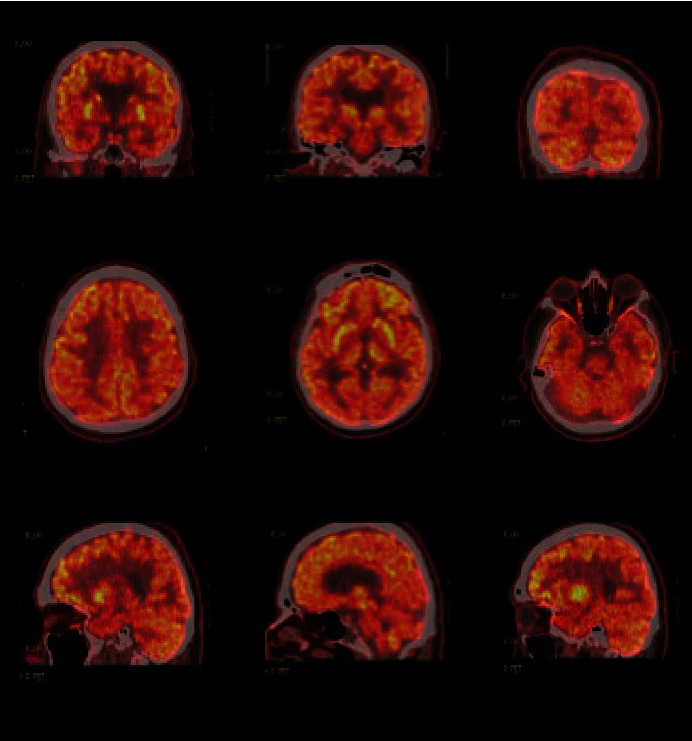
Fluorodeoxyglucose-positron emission tomography (FDG-PET) images of the patient demonstrating hypometabolism of the occipital and temporal lobes. Additionally, demonstrating the cingulate island sign (CIS): spared normal level of metabolism in the cingulate gyrus.
